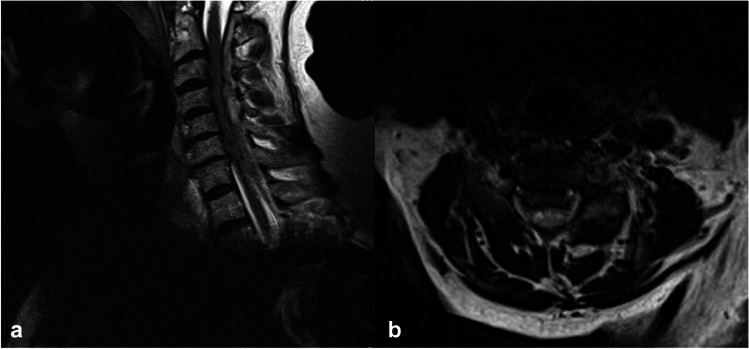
An 81-year-old man suffered from a sudden-onset of posterior neck pain and then, he developed paraplegia and anesthesia below T4. On sagittal T2-weighted images (a), the hematoma was located on the portion dorsal to the dural sac on the cervical spine (C3–C6). On the axial T2-weighted image (b), the hematoma was located on dorsal portion around to dural sac, and the dural sac was severely compressed
